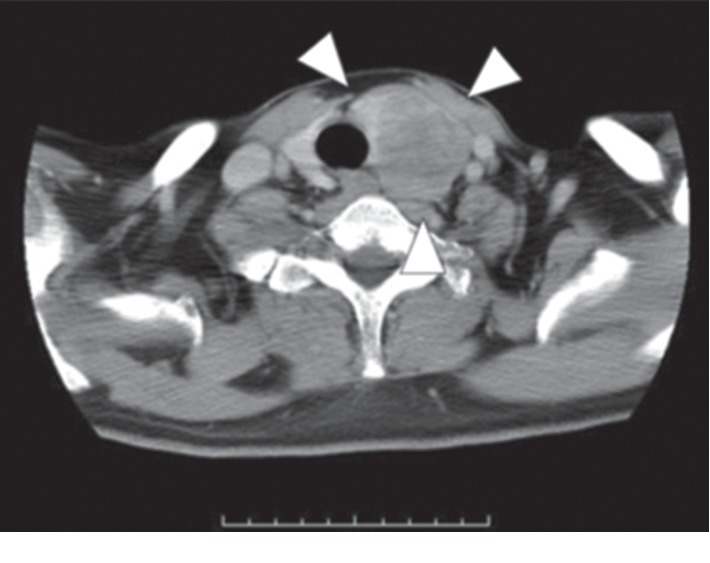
Imaging studies before the thyroidectomy. Contrast-enhanced CT showed a tumor in the left lobe of the thyroid.
